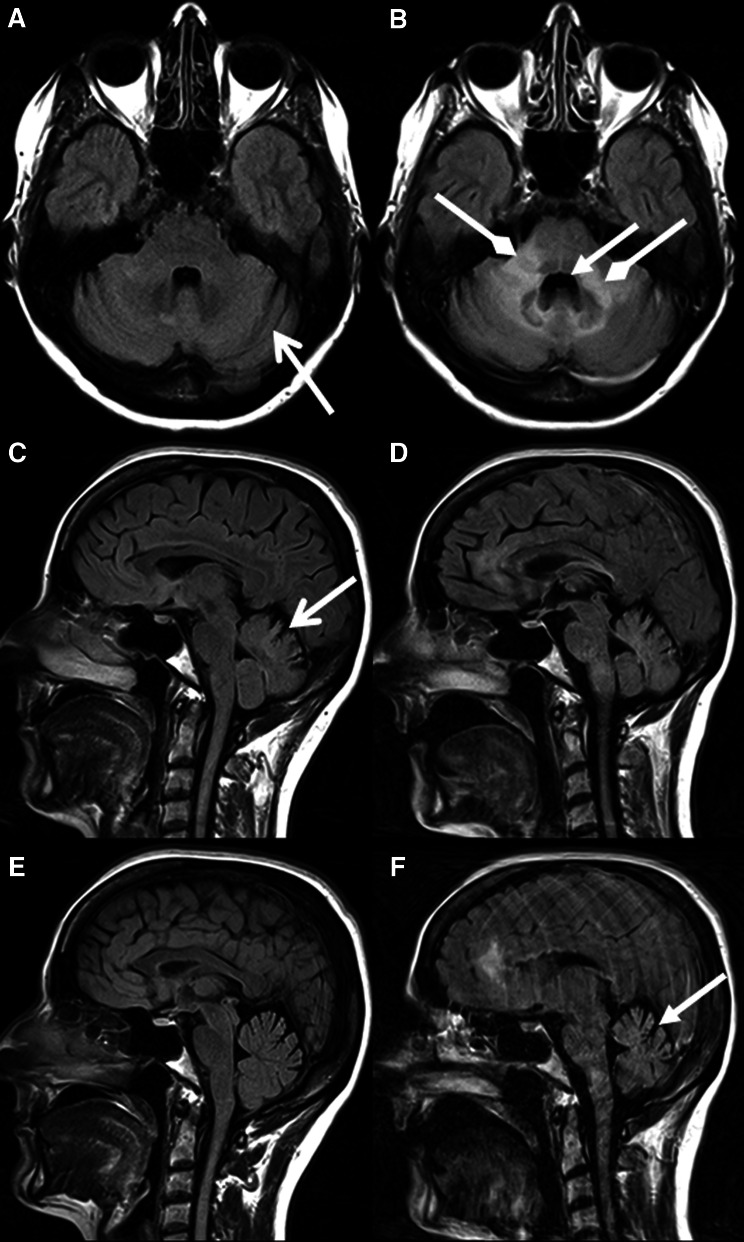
Axial fluid attenuated inversion recovery (FLAIR) images (a, d), and sagittal FLAIR images (b, c, e, f) of patient 3 showing grade 1 cerebellar atrophy at the time of PML diagnosis (a–c) and grade 2 cerebellar atrophy 3 months later (d–f). There were already bilateral dilated sulci at diagnosis (*open arrowhead*). However, there was clear loss of cerebellar volume 3 months later. Again, note the enlarged fourth ventricle (*closed arrowheads*). In addition, large hyperintense lesions in the cerebellar peduncles and brainstem developed (*diamond arrowheads*)
